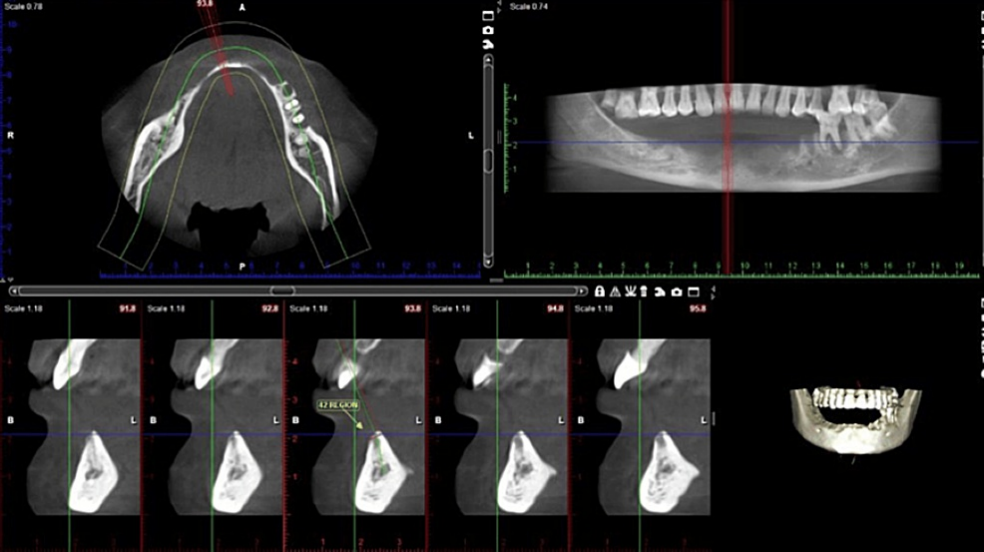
CBCT for implant planning CBCT: Cone beam computed tomography
